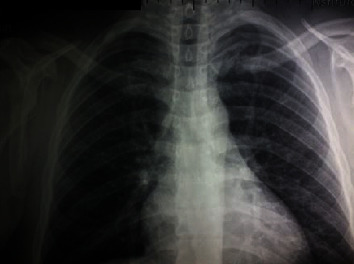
Anteroposterior chest radiography performed at the emergency department, showing fracture of the medial ends of both clavicles.
